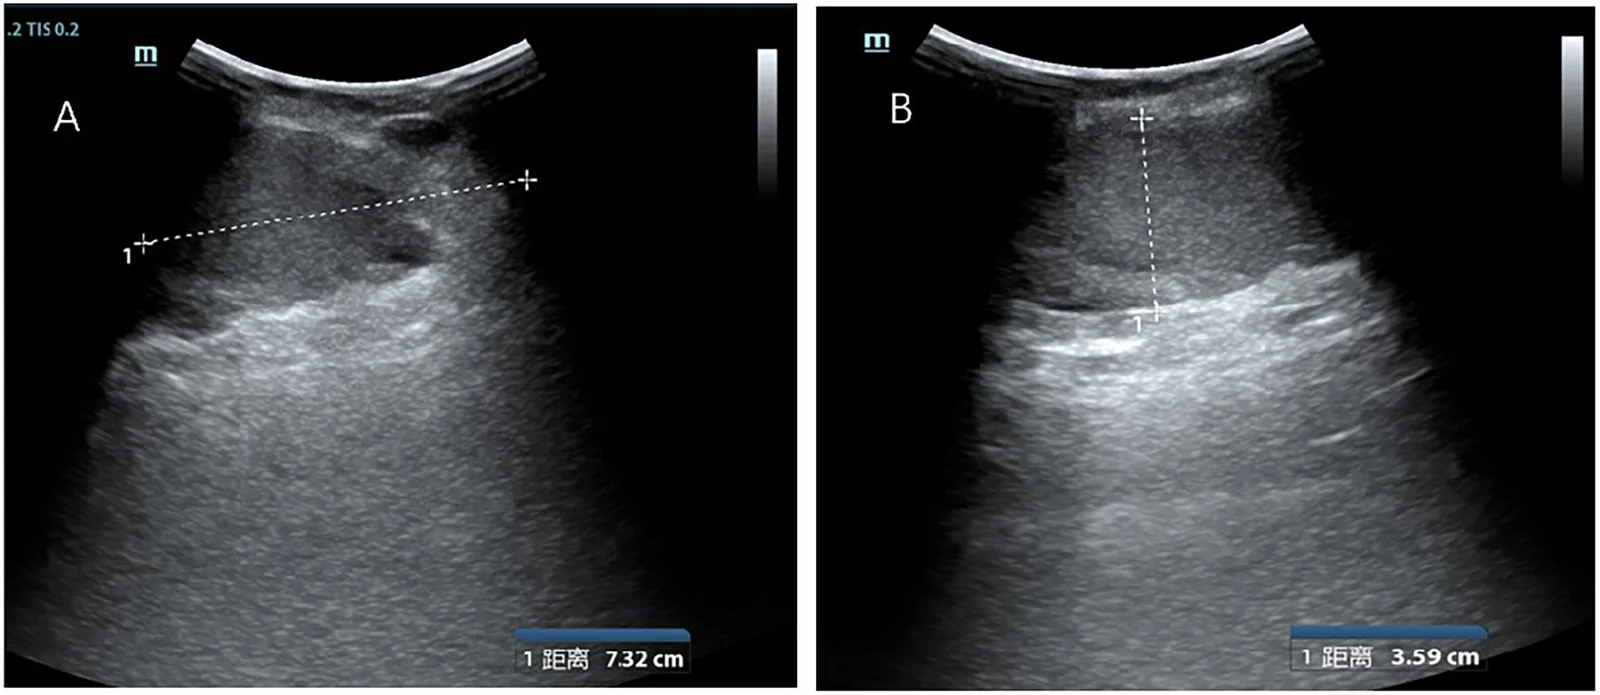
Initial sonogram of hematoma.
